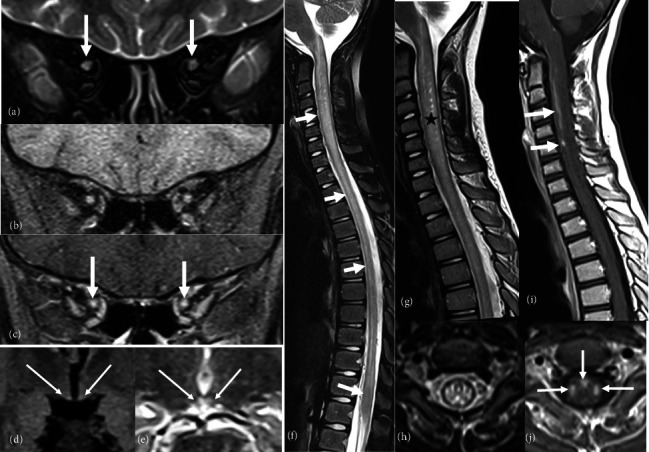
Radiological findings. (a–c) Coronal T2 (a), T1 with fat saturation (b) and T1 with fat saturation after gadolinium administration (c) weighted images on optic nerves. Bilateral increased T2 signal in the intra orbital optic nerves with enhancement (arrows). (d, e) Coronal fluid-attenuated inversion recovery (d) and T2 (e) weighted images. Bilateral hyperintensities involving the mammillary bodies (thin arrows). (f–h) Sagittal (f, g) T2-weighted images on the spinal cord. Hyperintense extensive lesion of the spinal cord from the bulbomedullary junction to the medullary cone (short arrows) with cervical spinal cord swelling (asterisk). (h) Axial T2-weighted image on the spinal cord showing the bilateral and symmetrical aspect of the hyperintensities which are selective, involving anterior, lateral and posterior columns. (i, j) Sagittal (i) and axial (j) T1-weighted images after gadolinium administration. Lateral and anterior column enhancement (arrows).
